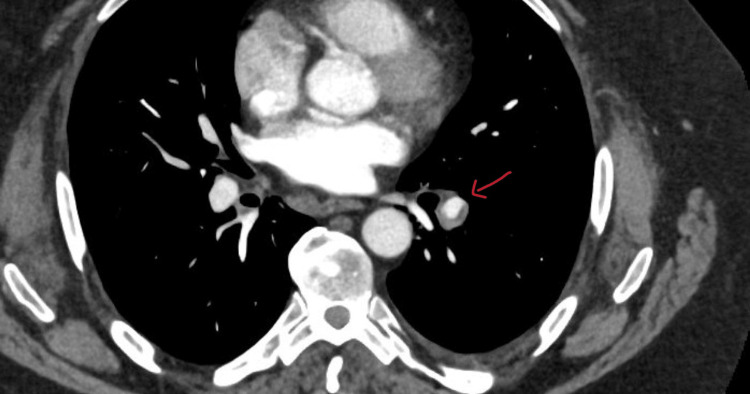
CT pulmonary angiography demonstrating an acute pulmonary embolism at the bifurcation of the left lower lobe pulmonary artery
